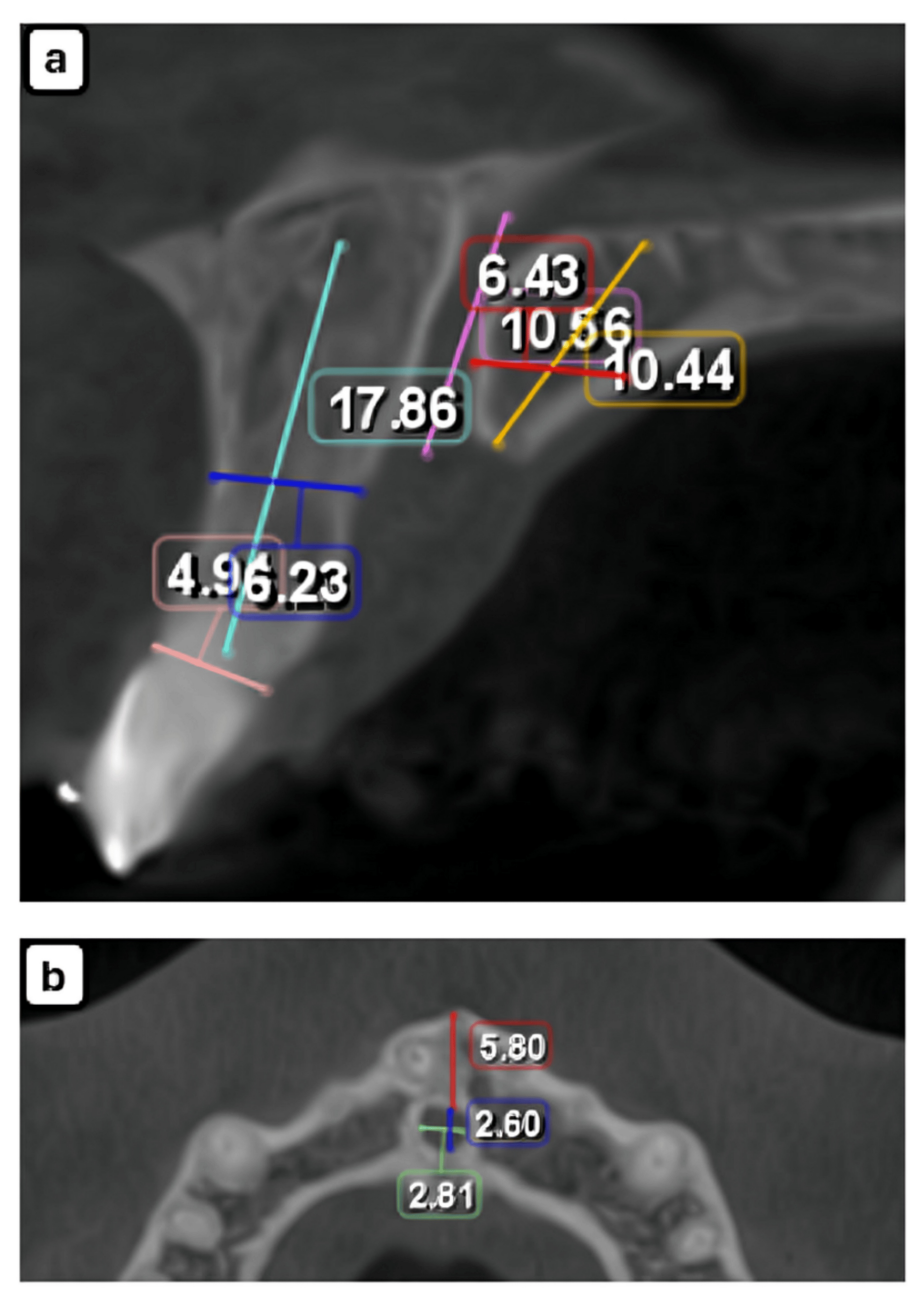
Anatomical parameters assessed on CBCT. Measurements performed on sagittal view (a) and axial view (b). Sagittal view: purple represents the length of the incisive canal. Yellow indicates the palatal bone length. Red marks the width of bone posterior to the canal. Blue denotes the width of bone anterior to the canal. Light blue shows the incisor root length measured from the cementoenamel junction to the apex. Pink refers to the incisor root width at the cementoenamel junction. Axial view: green represents the mesiodistal diameter of the incisive foramen. Blue indicates the labiopalatal diameter of the incisive foramen. Red shows the distance from the incisive foramen to the alveolar crest.
